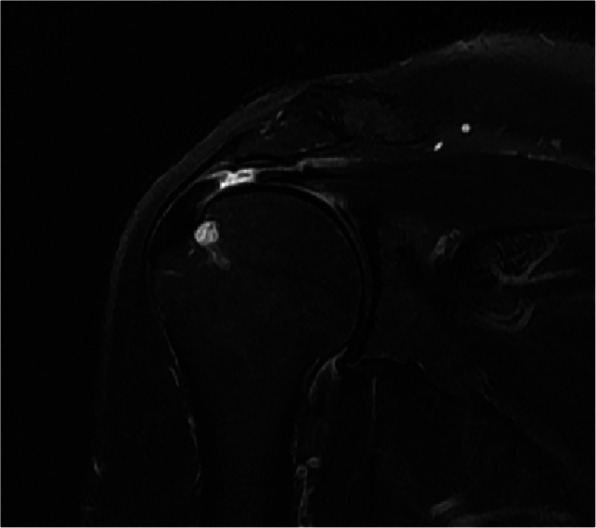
Preoperative T2-weighted fat-saturated magnetic resonance image showing a transtendinous rotator cuff tear with substantial remnant tissue attached to the footprint
